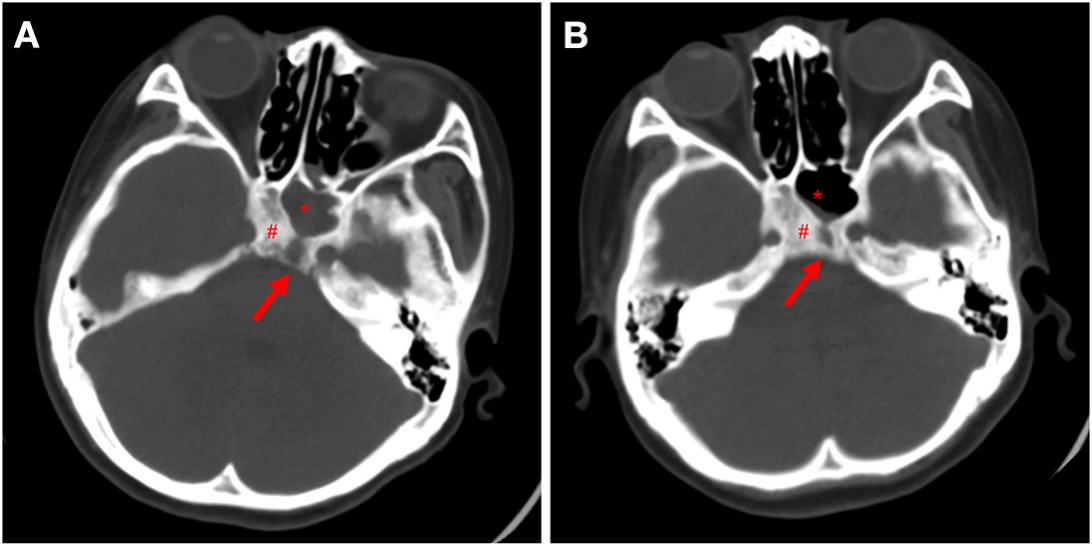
Paranasal sinus caused significant bony erosion. (A) Cranial CT at admission. (B) Cranial CT 1 month later. Red arrows indicate bone destruction. ^#^refer to the clivus, *for sphenoid sinus.
